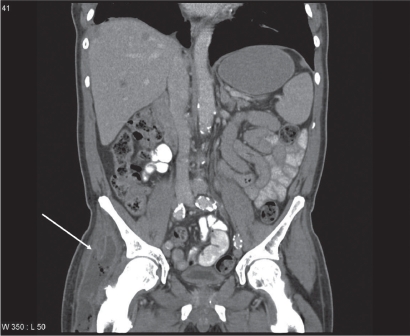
Contrast-enhanced computed tomography scan of the right hip after iv contrast injection: abscess indicated by white arrow.
